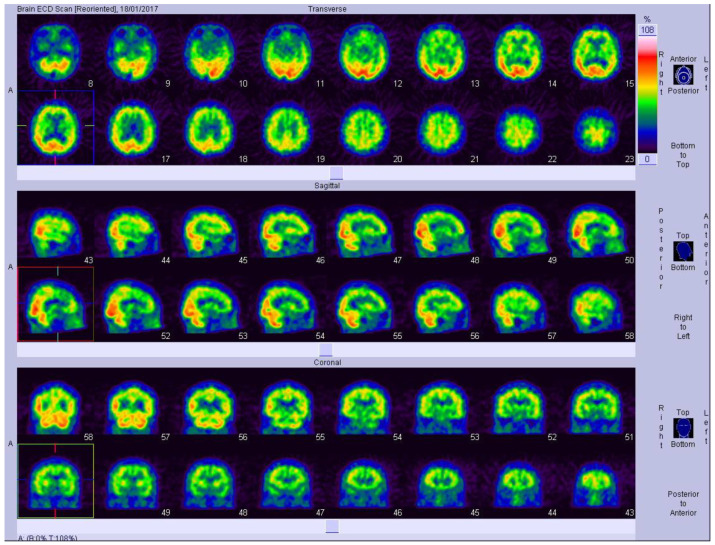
Single-photon emission computed tomography (SPECT) images of one participant. A total of 16 SPECT images were obtained in one section of one patient. Next, 48 SPECT images were obtained in three sections of this participant. A total of 4752 (48 × 99) SPECT images of all 99 participants were used. Of the 4752 images, 41 images beyond the area of brain were deleted. Therefore, 4711 images were used for further analysis. The colors in the figure represent the ranges of blood flow with red being the most blood flow and violet the least.
